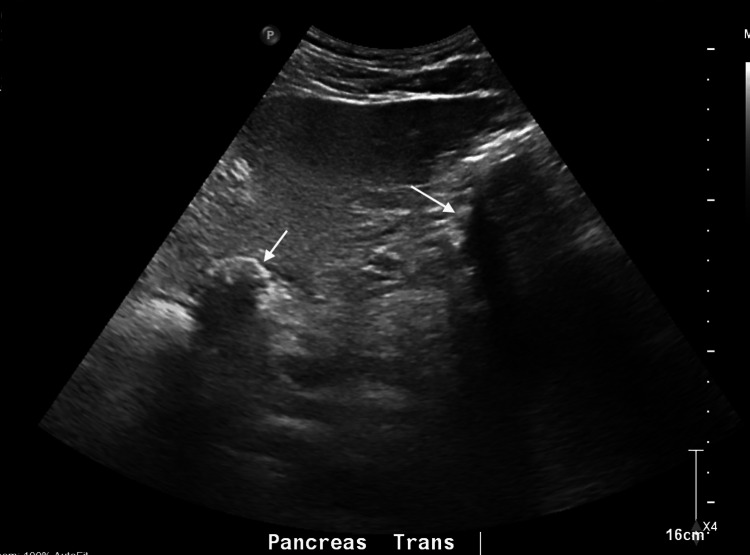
Ultrasound of the RUQ of the abdomen. There are stones (white arrows) with acoustic shadowing within the gallbladder and a fatty liver. RUQ: right upper quadrant.
